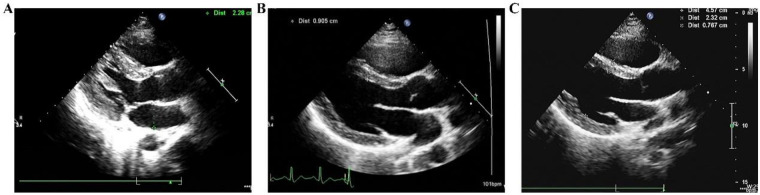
Serial echocardiograms at different periods showing no abnormal intracardiac masses. (A) August 31, 2022; (B) November 22, 2022; January 10, 2023.
